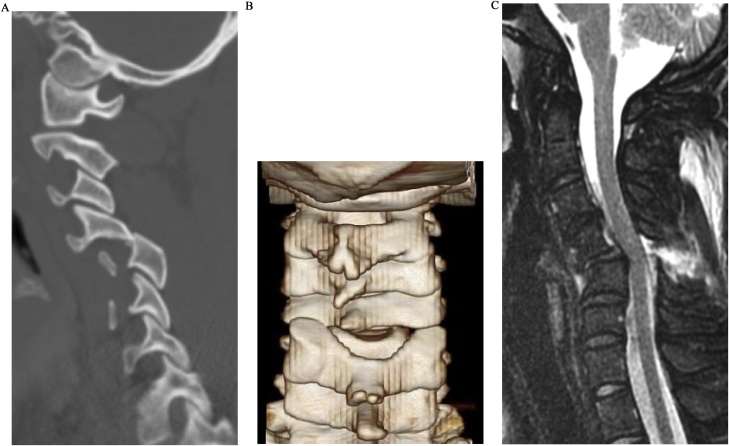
a) Computed tomography (CT) images showing left facet dislocation at C4-C5. b) Three-dimensional CT reconstruction of the cervical spines, showing a dilated interlaminar space. c) Magnetic resonance images showing spinal cord compression at C4-C5 on T2 STIR-weighted sequence with no obvious disc herniation.
